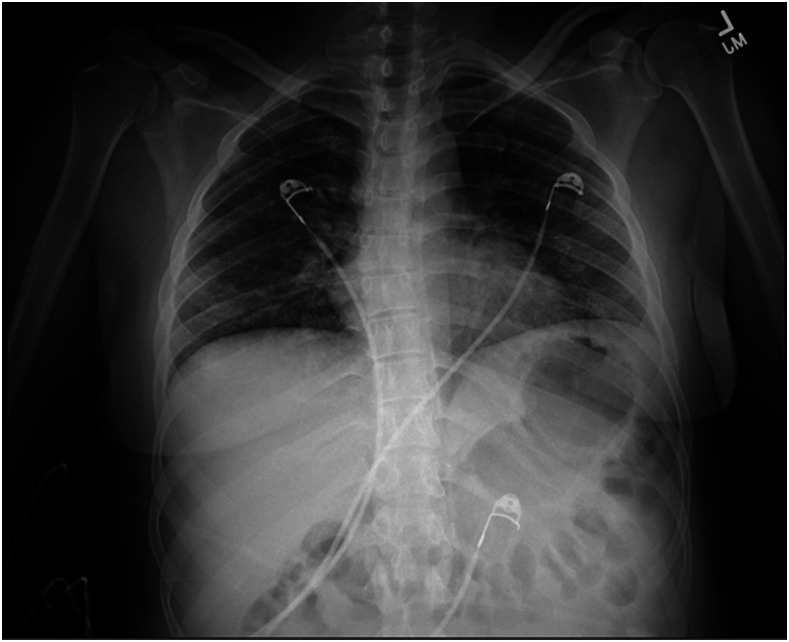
A portable chest X-ray shows a normal cardiac silhouette. There is no focal consolidation or effusion. Costophrenic angles are present. Trachea is midline.
